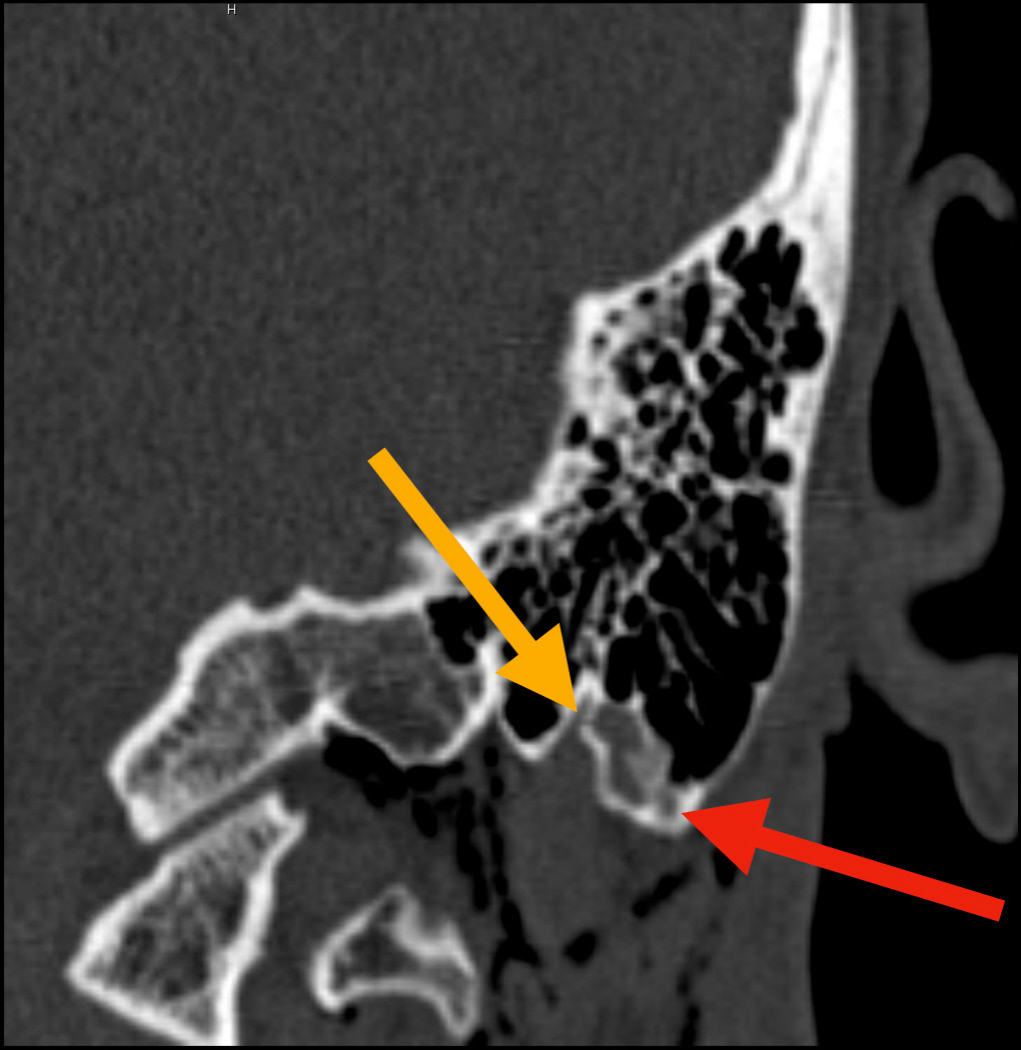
Coronal plane HRCT scan – Left side: medial mastoid apex fracture (yellow arrow), fluid within the mastoid air cells (red arrow), presence SCE at the level of C1, lateral to the occipital condyle
